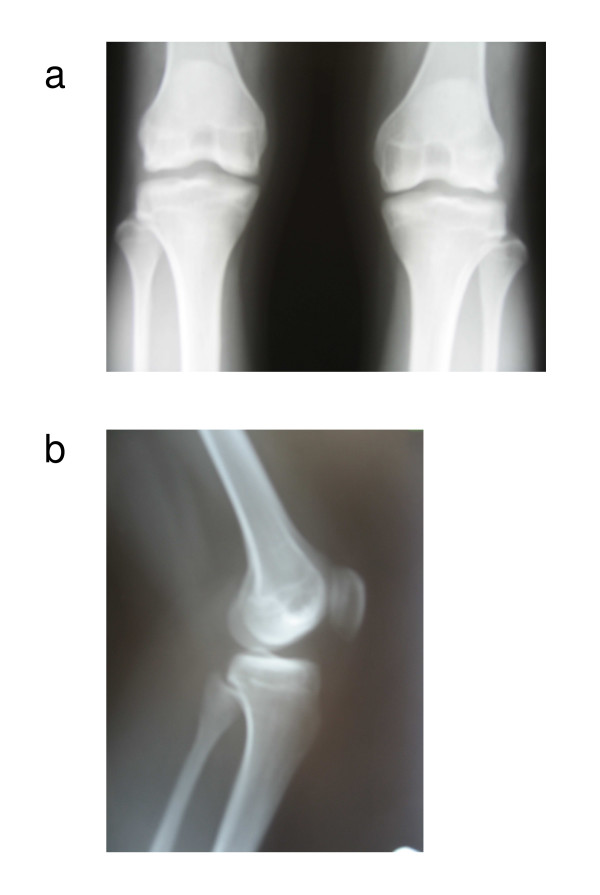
Radiograph of the knee. (a) Anterior-posterior view; (b) lateral view.
